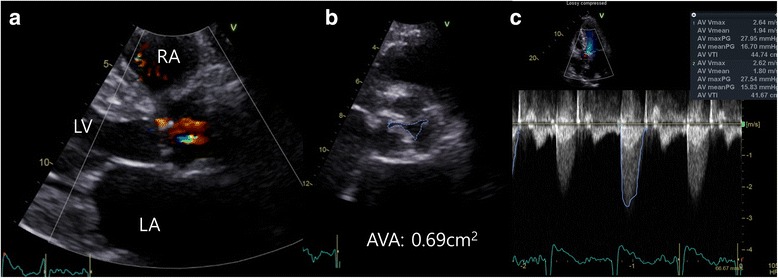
Two-dimensional echocardiography findings. a Before the TAVR procedure, echocardiography showed degenerative change in the aortic valve with severe calcification and left ventricular hypertrophy. b The aortic valve area was 0.69 cm^2^ based on 2D planimetry. c The mean systolic pressure gradient was 16.3 mmHg and aortic valve area was 0.86 cm^2^ based on the continuity eq. RA: right atrium; LV: left ventricle; LA: left atrium
